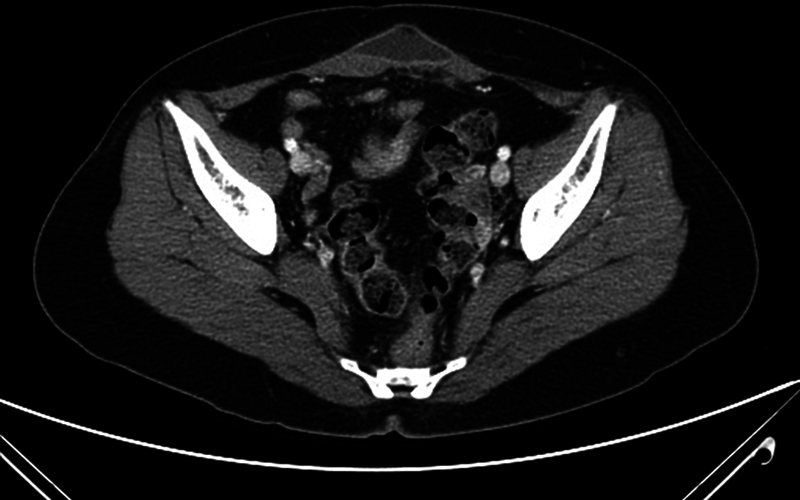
Axial contrast-enhanced CT image of case 3 showing abdominal wall hernia without overlying skin injury after HIFU therapy. Rectus abdominis muscle injury and the overlying fluid collection lasted for more than 1 year in spite of repeated needle aspiration. CT, computerized tomography; HIFU, high-intensity focused ultrasound.
